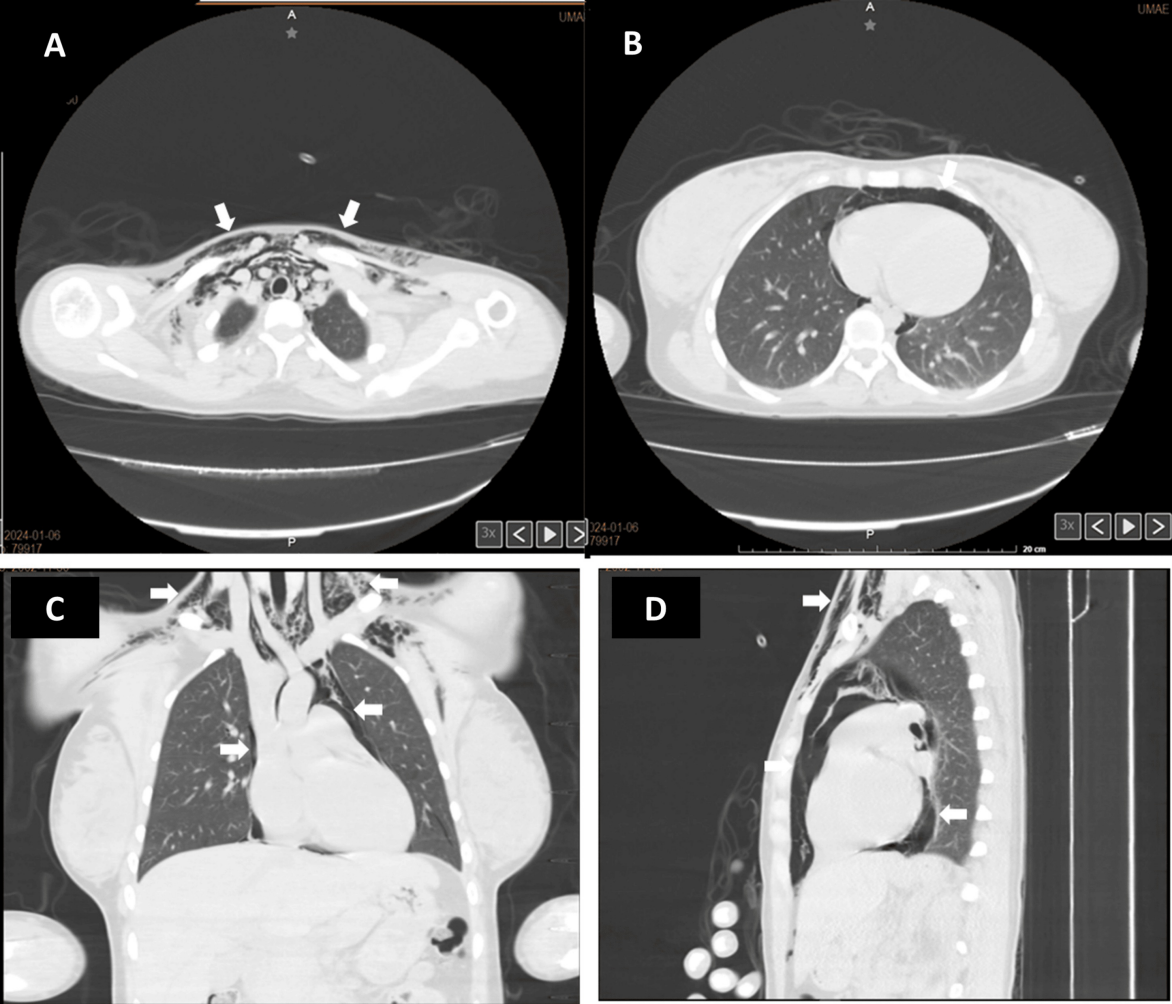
Non-contrast chest CT (lung window) on day one Different planes, i.e., axial (A and B), coronal (C), and sagittal (D) showing subcutaneous emphysema extending to the soft tissue of the supraclavicular region and neck (A and C) and in the anterior sternal region (D) along with evidence of pneumomediastinum (B, C and D). CT: computed tomography
